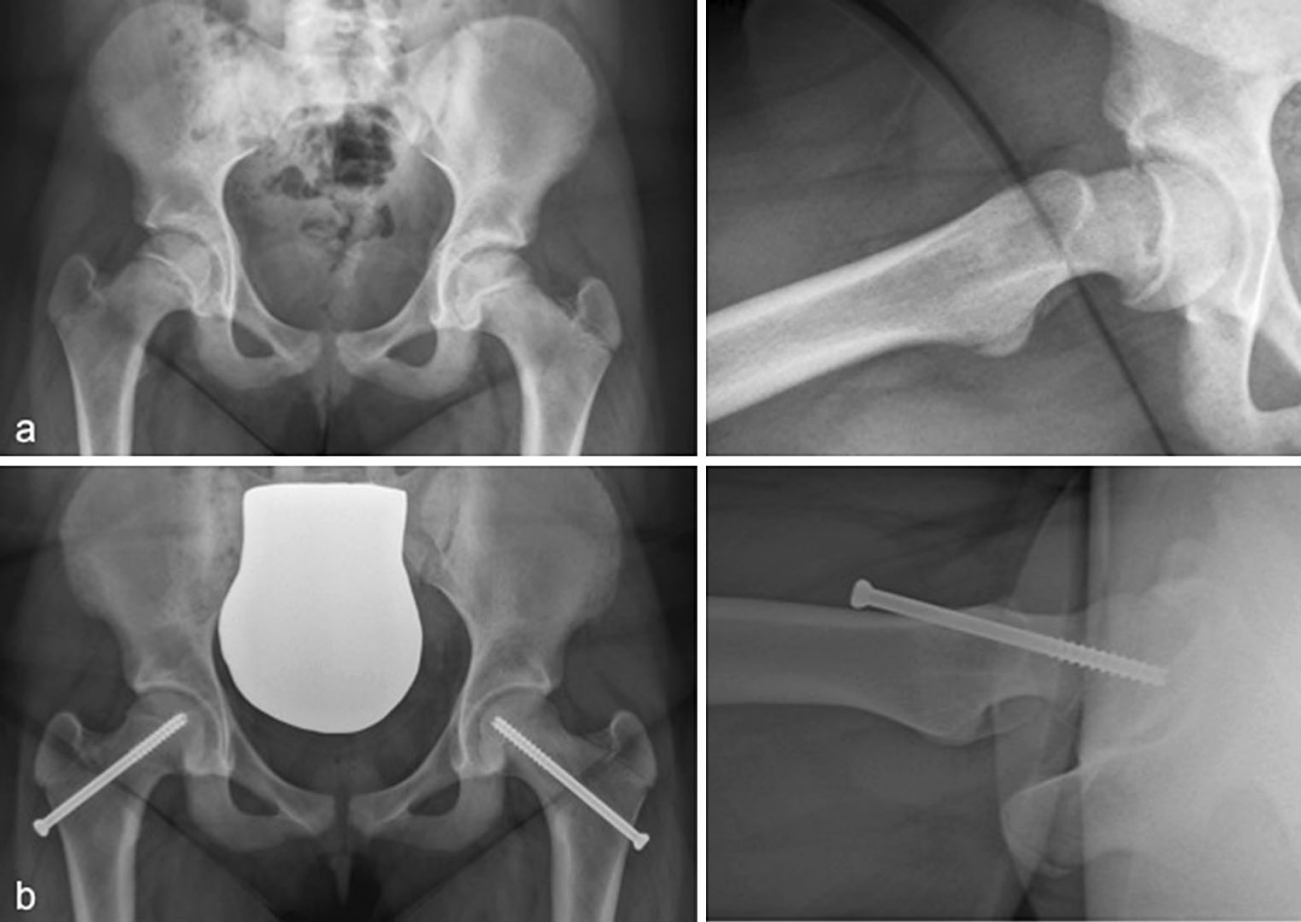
Antero-posterior and frog-leg radiograph prior (a) and after (b) in situ fixation and arthroscopic osteochondroplasty of the femoral neck (patient no. 13)
